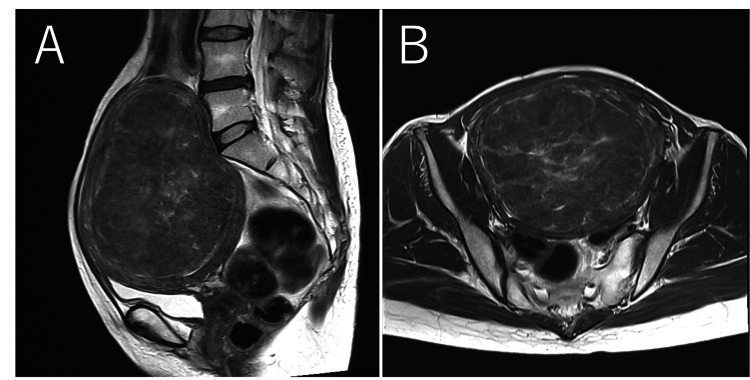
Magnetic resonance imaging (MRI); (A) sagittal T2-weighted image, (B) axial T2-weighted image. No abnormalities were observed in the uterus or adnexa apart from the leiomyoma.
